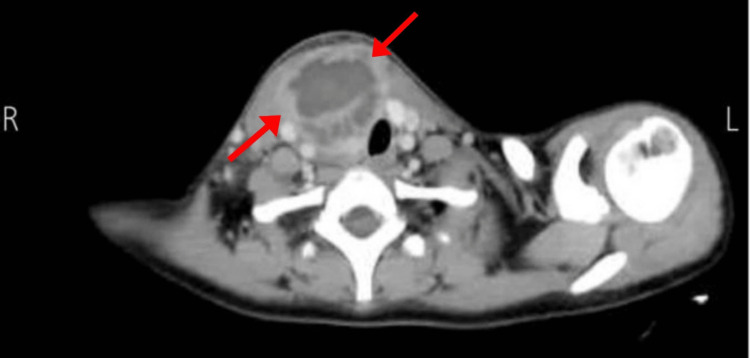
Neck CT scan showing the thyroid abscess (red arrows)
